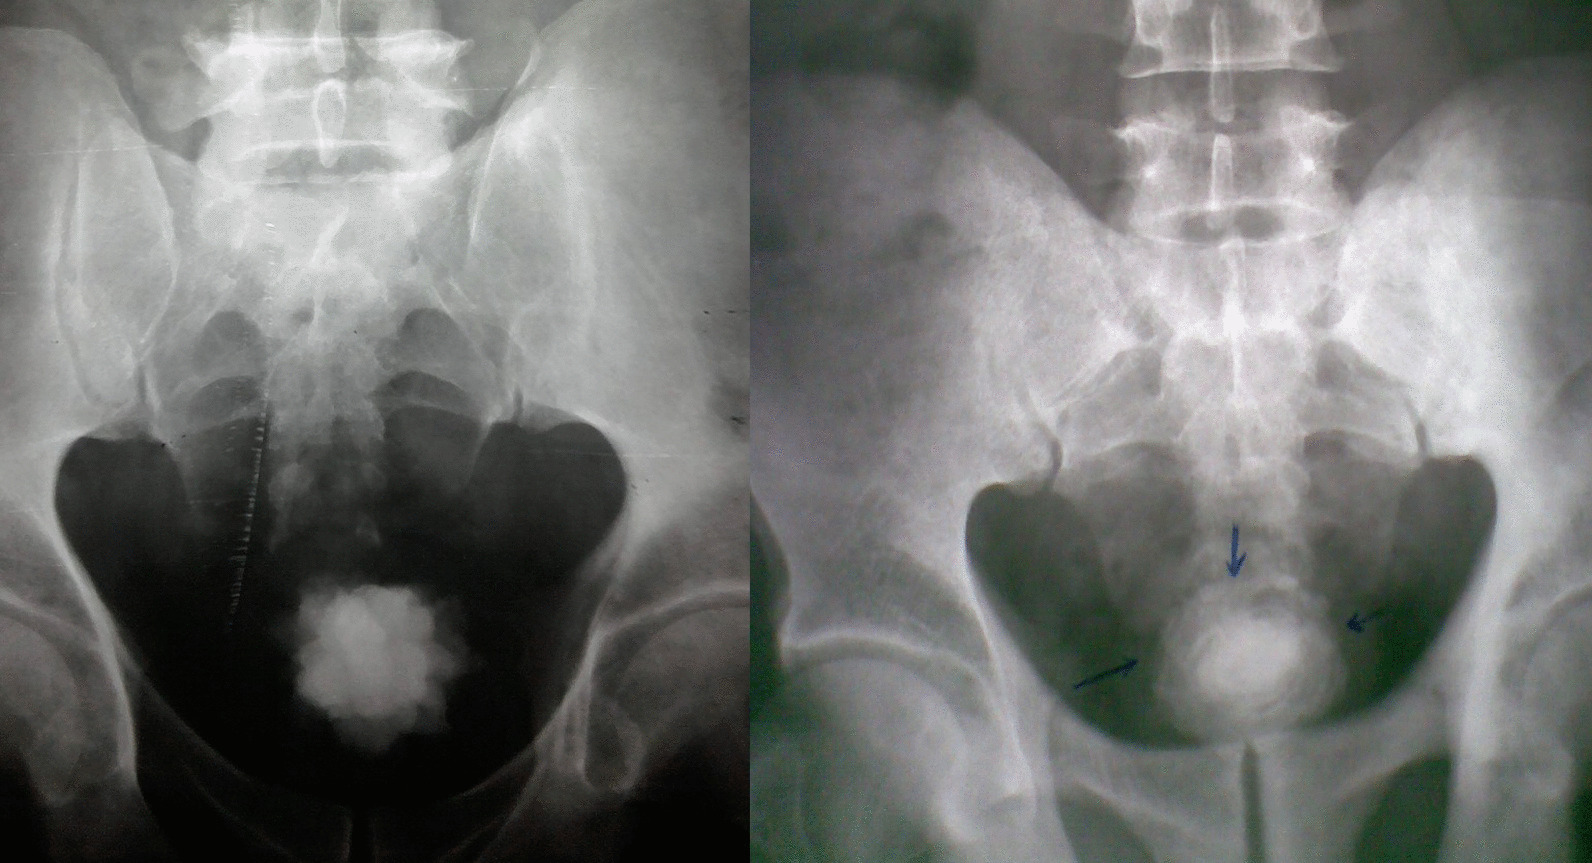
Giant stones of the urinary bladder with rounded shapes, rough surfaces, and moderate densities in plain radiographs: The left-sided one has a prominently serrated surface (a spiky stone) and the right- sided one has a less-prominently serrated surface
